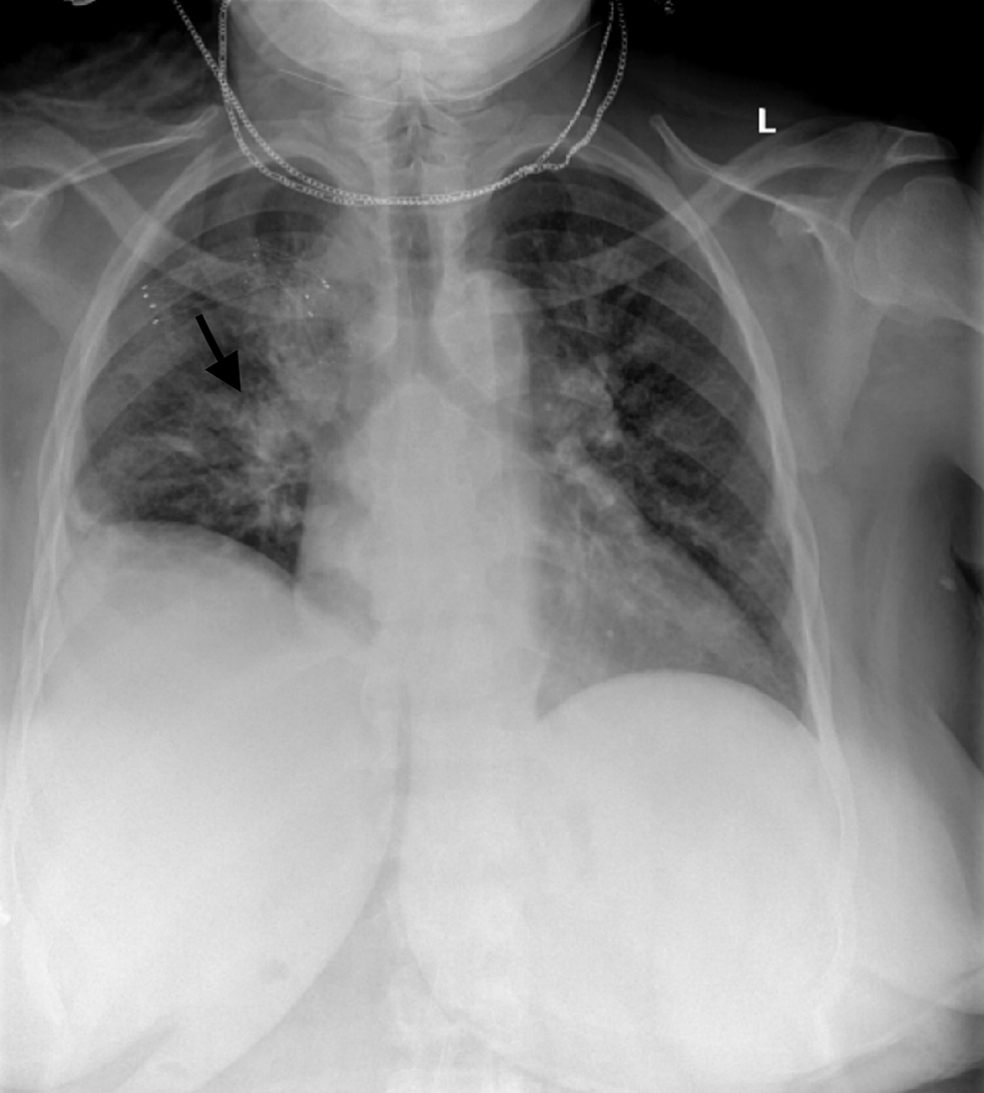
Chest X-ray (posteroanterior view) with right lung infiltrate (black arrow).
